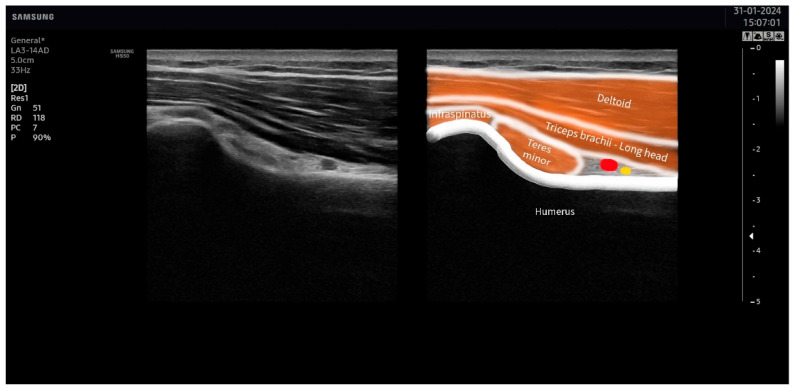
Ultrasound identification of the axillar nerve (in yellow) at the superior border of the quadrangular space under the area between the teres minor and the long head of the triceps muscles. The artery is identified in red.
